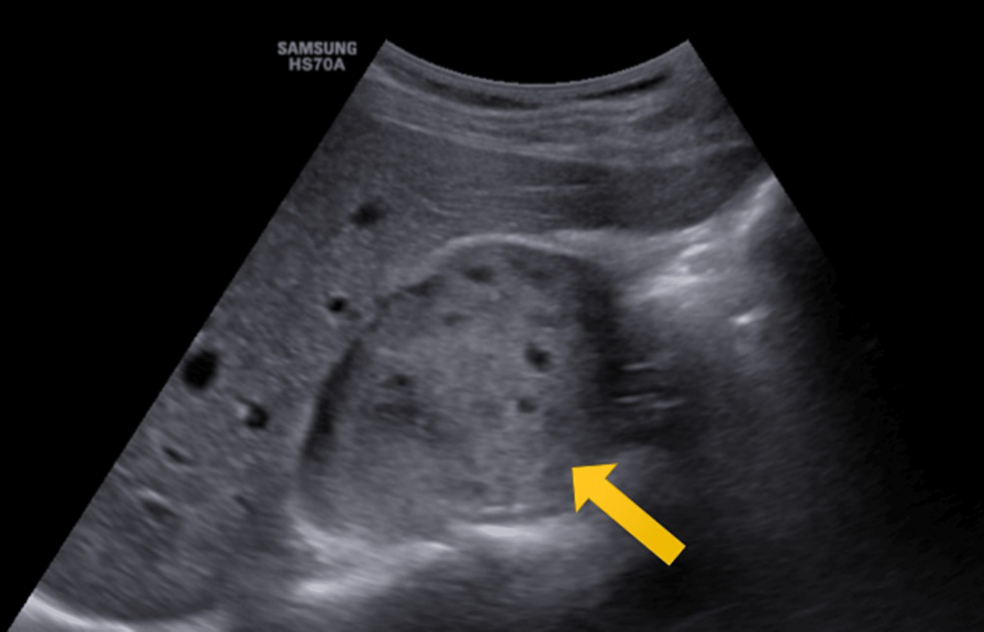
On B-mode ultrasound, an iso-hyperechoic mass in the right suprarenal region with multiple anechoic areas is shown (yellow arrow).
